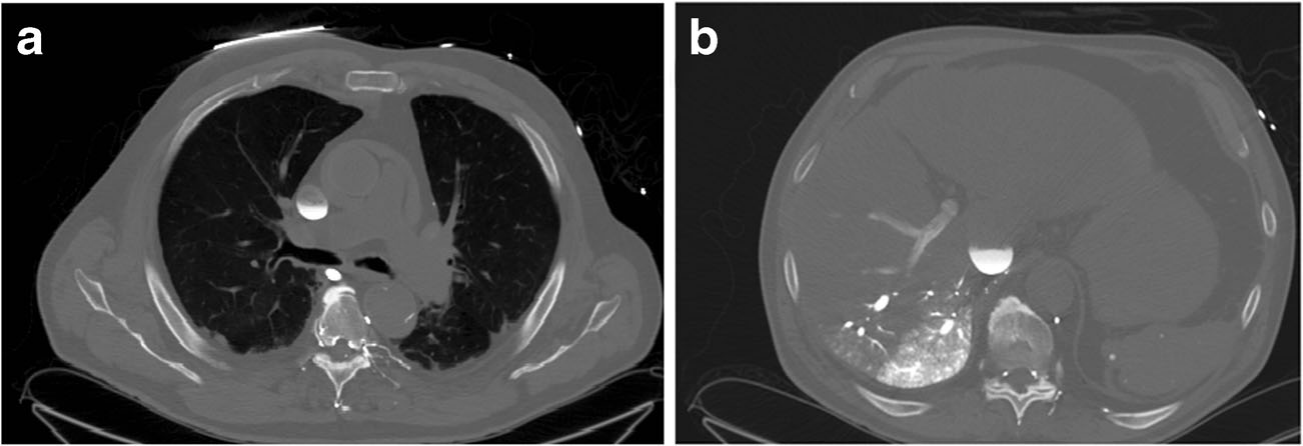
Asystole with no cardiac output. Patient with cardiac arrest at the time of CTA for acute aortic syndrome. Axial CTA at the level of the carina (a) shows no opacification of pulmonary artery, ventricles, or aorta. The CT technician subsequently called the radiologist to check the images and ask for a repeat injection after identifying abnormal contrast enhancement. Review of the axial images in the upper abdomen (b) reveal contrast reflux into IVC, dependent hepatic veins, and a blood-contrast level in the IVC. Contrast opacifies the right portal vein secondary to backflow from hepatic vein into portal vein. Contrast opacification is of the dependent vasculature only. This prompted initiation of cardiopulmonary resuscitation and calling the code team. See also Movie 1
